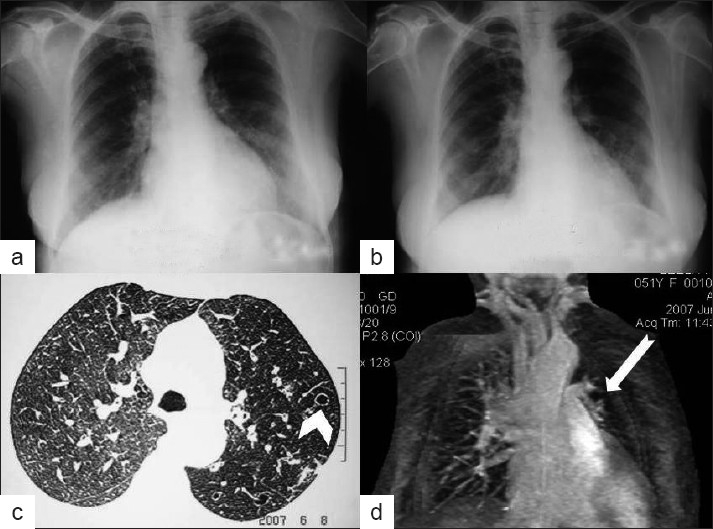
Inspiratory (a) and expiratory (b) chest radiographs of 51-year-old female showing small hyperluscent left hemithorax with air trapping on expiration. HRCT (c) shows reduced volume of left lung with decrease in parenchymal attenuation and bronchovascular markings on left side. Bronchiectatic areas are seen in left hemithorax (white arrow head). MR angiography (d) shows typical pruned tree appearance on left side (thick white arrow)
